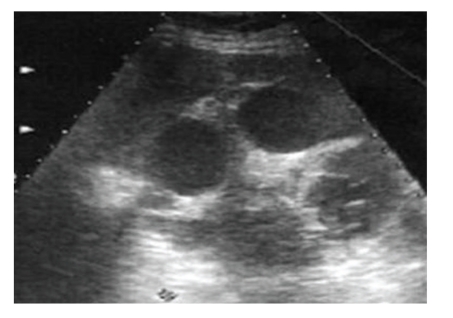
Ultrasonography revealed a multivesicular cystic mass of 12 × 11 cm, in the location of splenic hilus.
